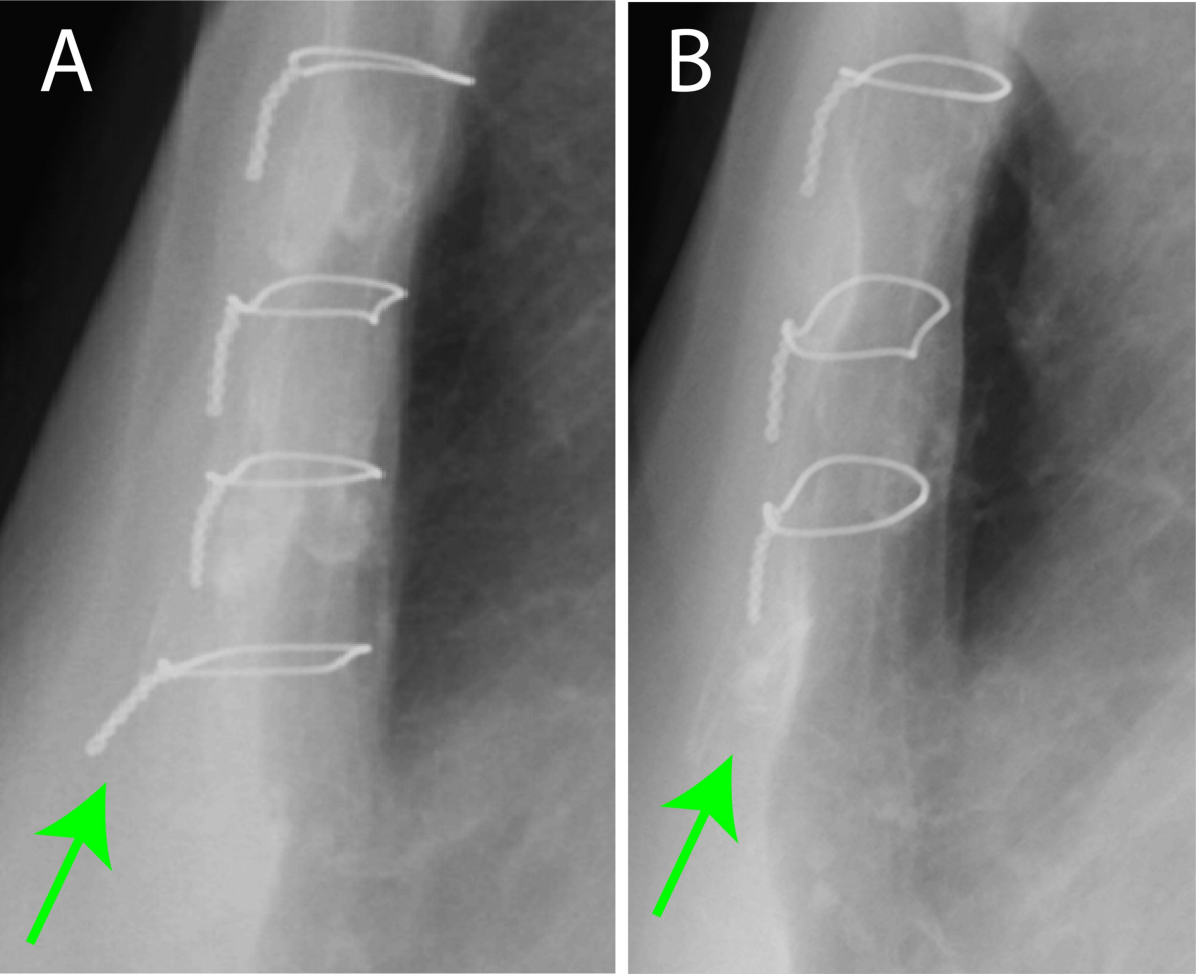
Chest X-p before and after wire removal (A) Before wire removal. Lateral chest X-p reveals the sixth wire was standing up. The angle between the vertical line is 39.0° (green arrow). (B) After wire removal. The sixth wire is removed (green arrow). Chest X-p: chest X-ray photograph
